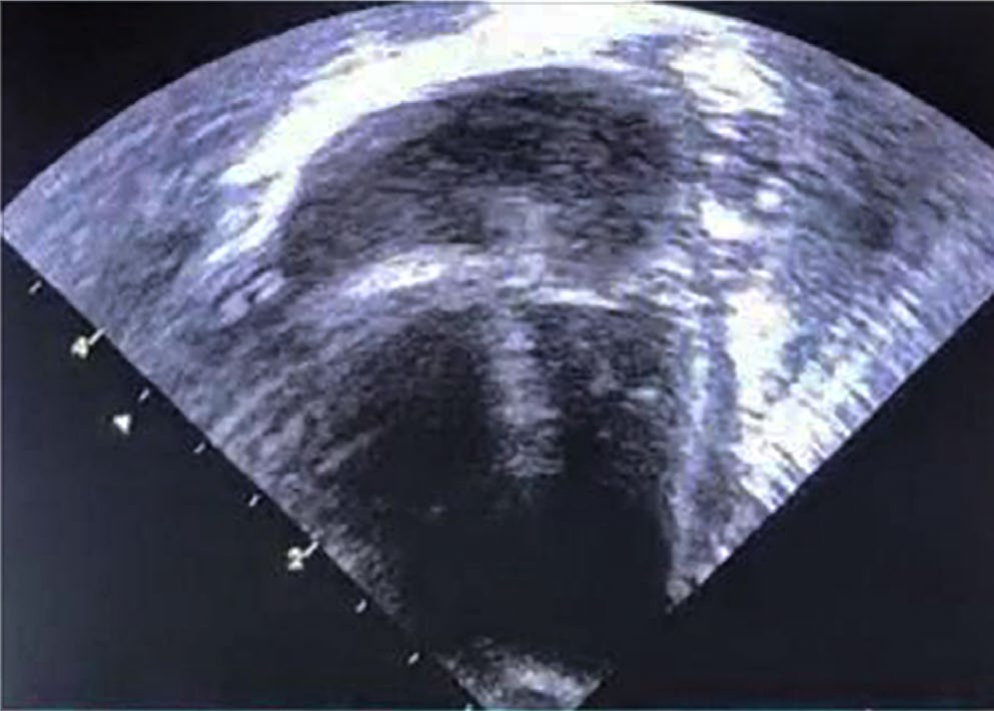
Echocardiography shows resolution of pericardial effusion
